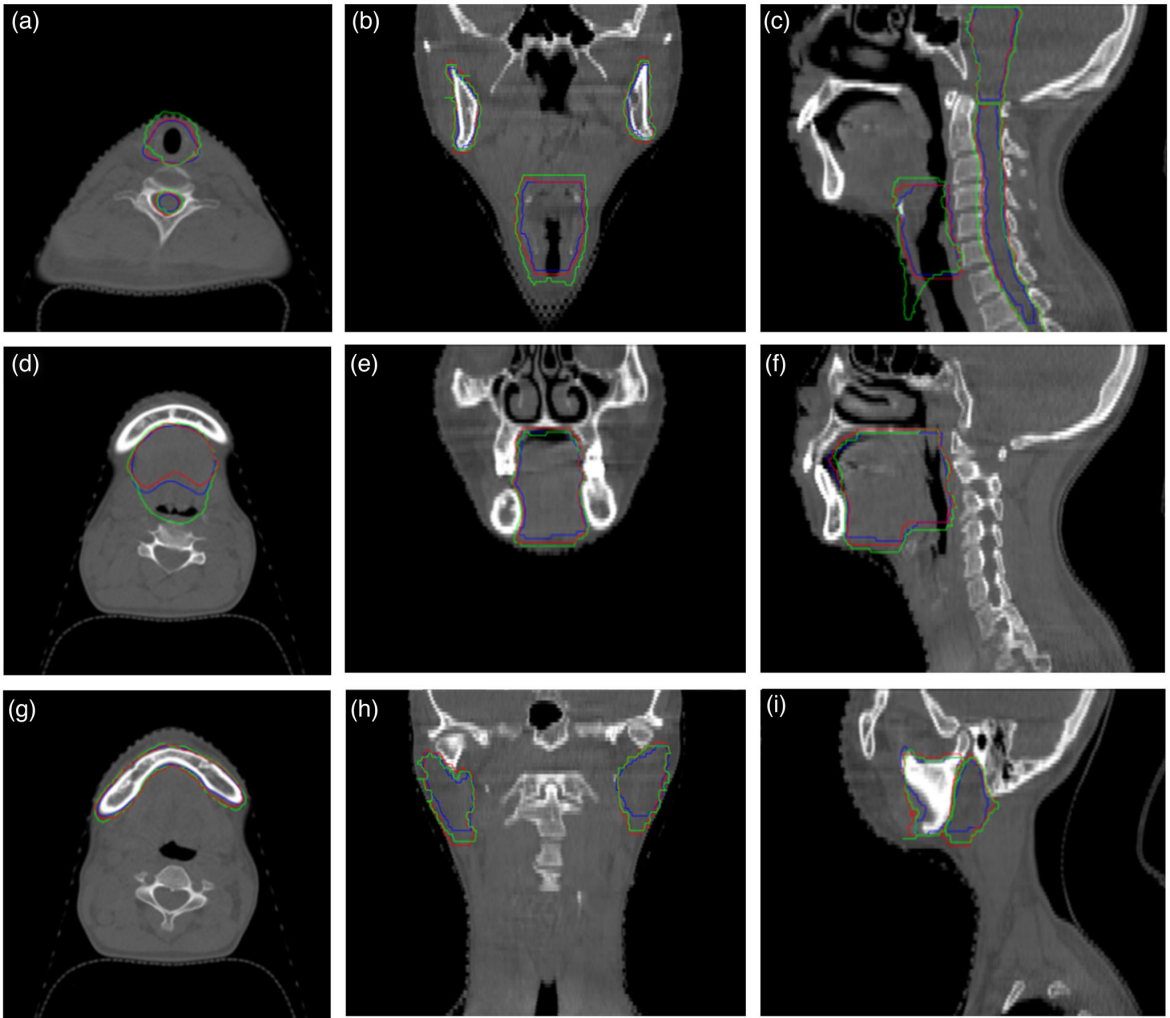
Examples of contour propagation using two deformable image registration (DIR) methods. The blue region is the delineation by cone‐beam computed tomography (CBCT), the red is warped organs at risk (OARs) generated by in‐house hybrid method, and the green is generated by NiftyReg. (a–c) The brain stem, larynx, and spinal cord results; (d–f) the oral cavity; (g–i) mandible, parotid
